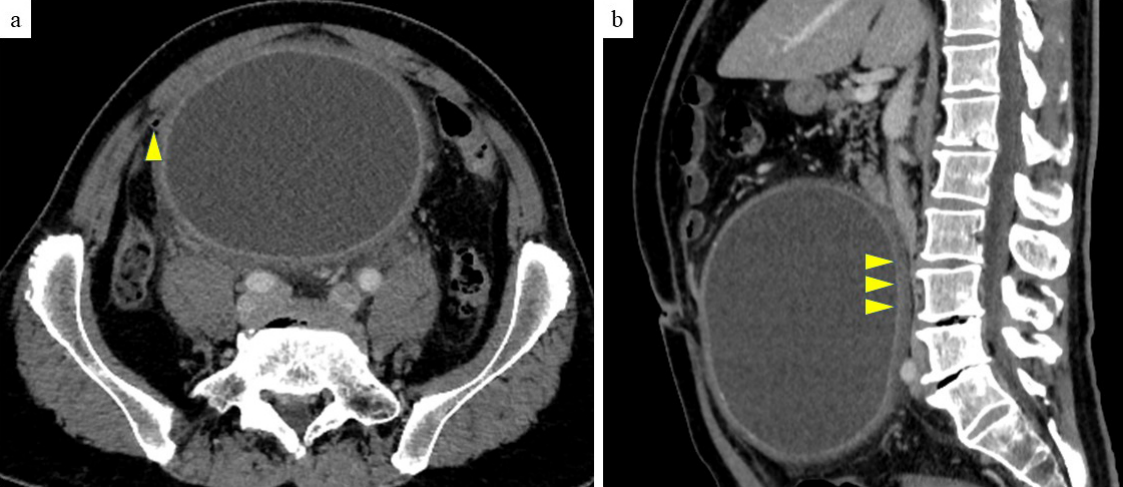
a) Contrast-enhanced horizontal CT image: The appendix is in contact with a large cyst (arrowhead). The continuity between the appendix and cyst is unclear on CT. b) Contrast-enhanced sagittal CT image: The IVC is significantly compressed by the large cyst (arrowhead). CT: computed tomography; IVC: inferior vena cava.
